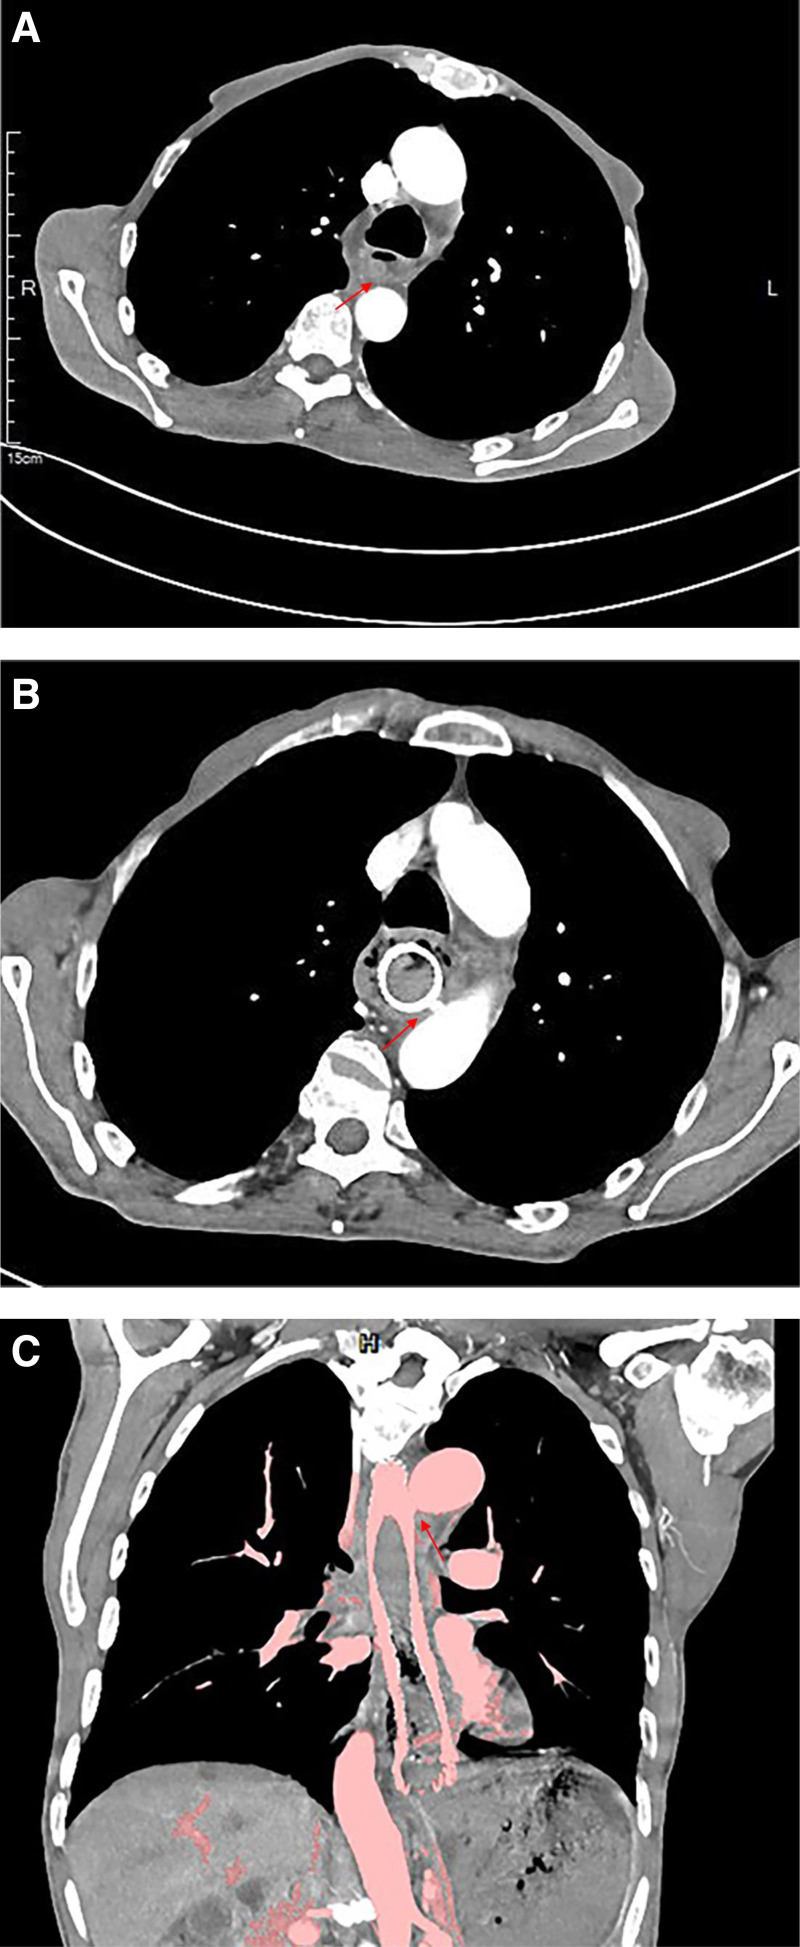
CT images of esophageal stent placement. (A) No false aortic aneurysm was detected at the indicated location prior to esophageal stent placement. (B) and (C) show arrows indicating the presence of a new pseudoaortic aneurysm following esophageal stent insertion. CT = computed tomography.
